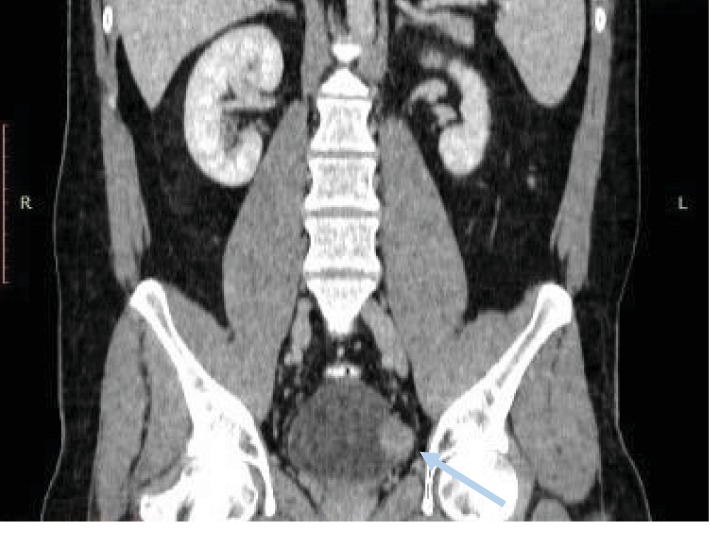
Bladder leiomyoma (grey arrow). Computed tomography, abdomen. Coronal view.
